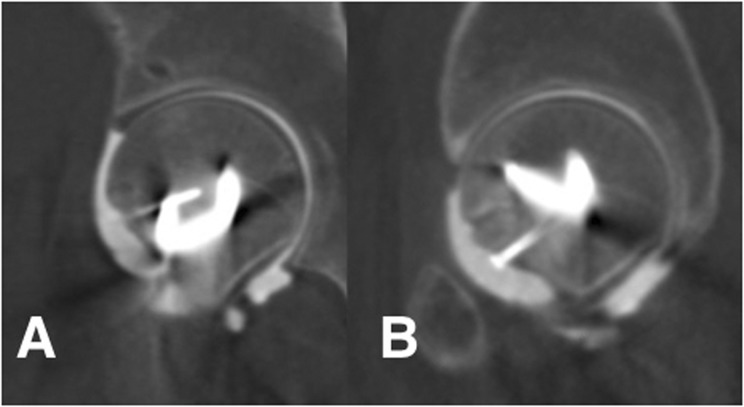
CT arthrogram showing site of graft with full consolidation and no residual defect (A) as well as adequate sphericity in the most anterior part of the FHNJ in a sagittal view (B).
